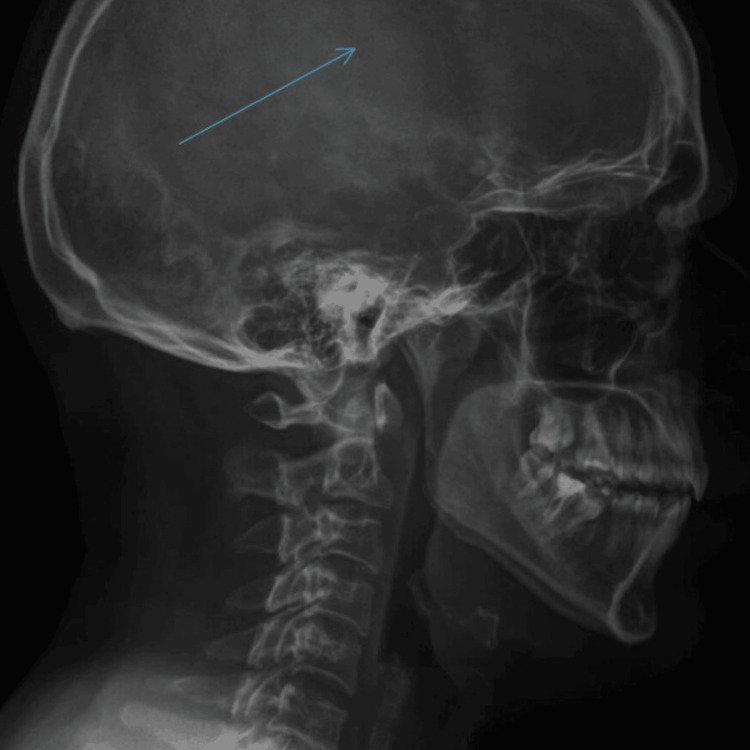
A Lateral skull radiograph demonstrates preserved calvarial contour and intact skull base.
